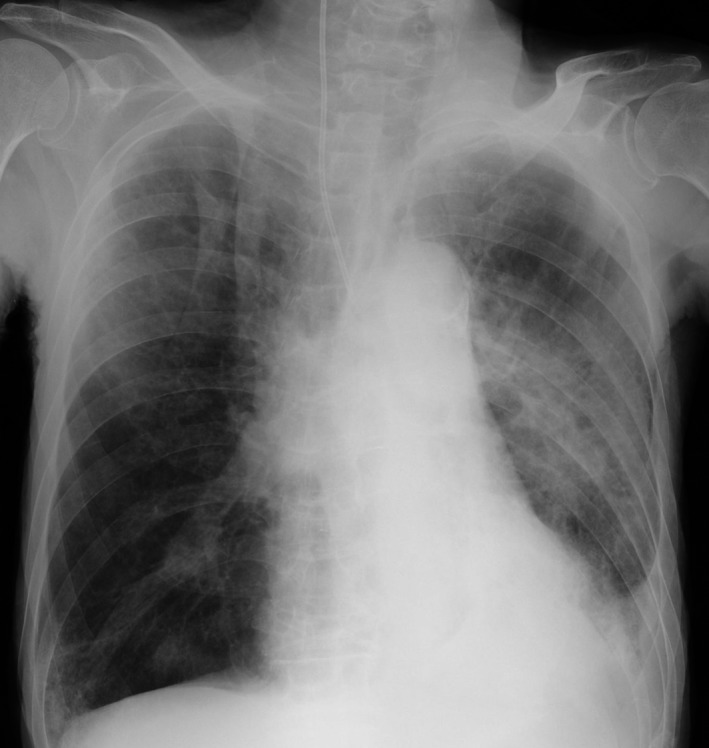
Chest radiograph before transfer to the Department of Internal Medicine
